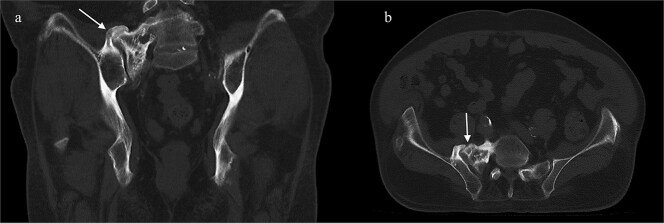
Coronal CT pelvis (left) and axial (right) demonstrated hyperostosis along the right SI joint suggestive of an osteoblastic lesion between the L5 transverse process and sacral ala (arrows).
